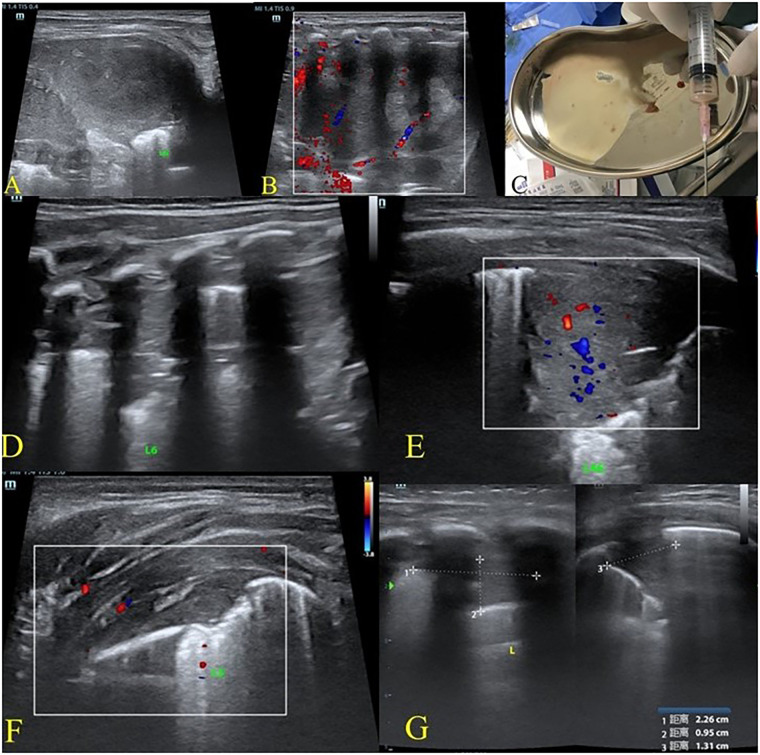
Ultrasound-guided diagnosis and drainage of an enlarged left lower lobe pulmonary abscess on hospital day 14. On hospital day 14, without ventilatory support, ultrasound showed persistent left lung consolidation with a markedly enlarged abscess (A,B). Under ultrasound guidance, percutaneous aspiration and closed thoracic drainage of the left lower lobe abscess were performed, and 10 mL of thick gray pus was aspirated (C) On hospital day 29, without ventilatory support, ultrasound demonstrated reduced consolidation in the left lung with complete resolution of the abscess (D,E). Mild pleural thickening was observed on the left side (F) Followup ultrasound after discharge showed a small residual consolidation in the left lower lobe without evidence of abscess (G).
